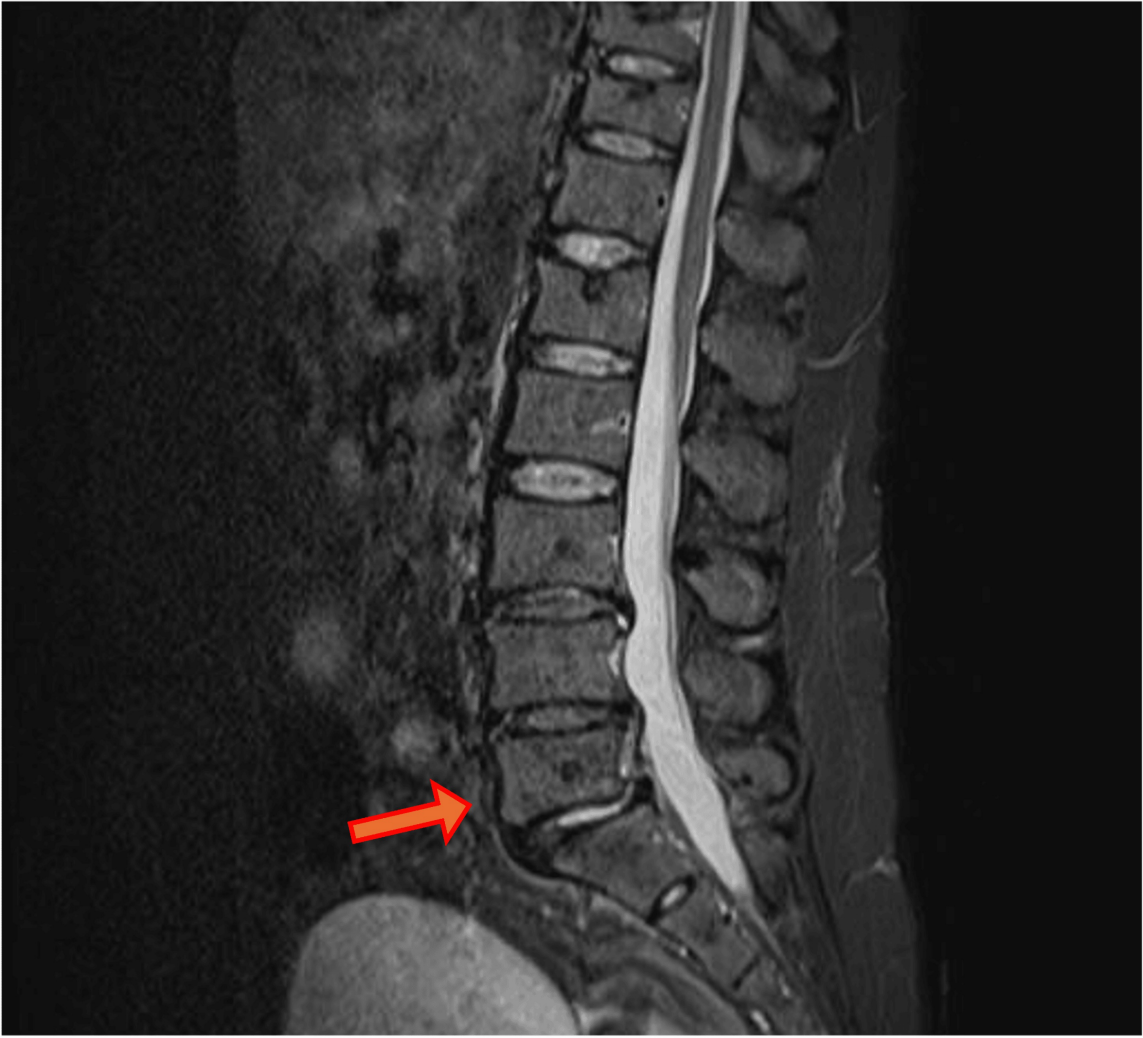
MRI STIR at the patient's first presentation In MRI STIR, compression fractures at L1 and spondylolisthesis at L5 (red arrow) are observed. No obvious abscesses surrounding the vertebral bodies are noted. STIR: short tau inversion recovery
